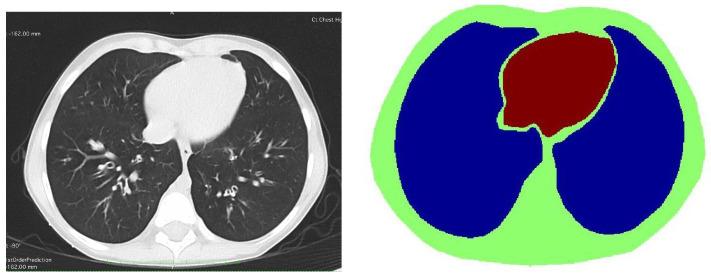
One slice of the CT scan of the subject and the static prior constructed from the CT image.
